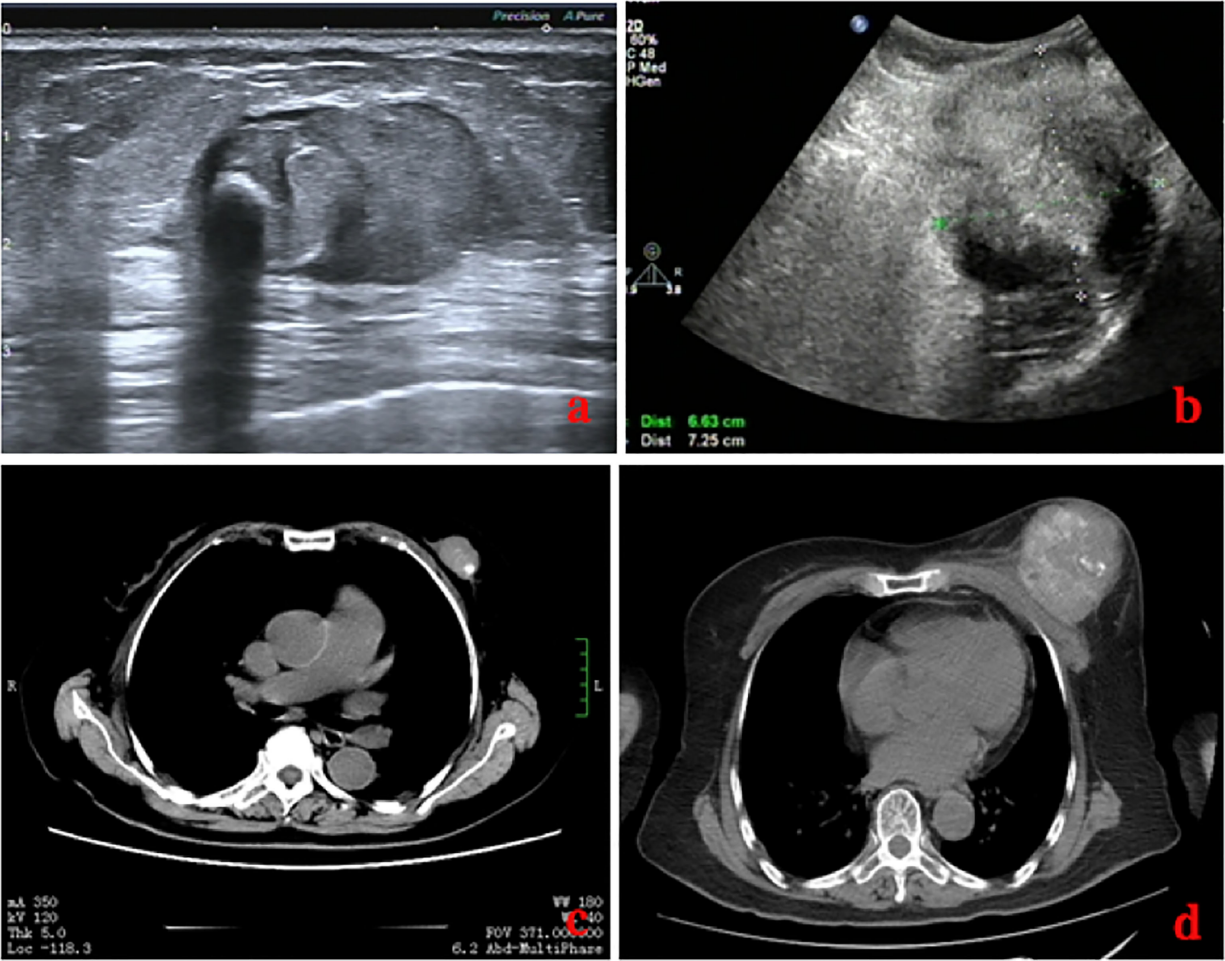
Comparison of images on US (A, B) and CT (C, D) of the patient before and after 8 months. Eight months later, the lesion was much larger with unclear boundaries, and there were much more calcifications.
